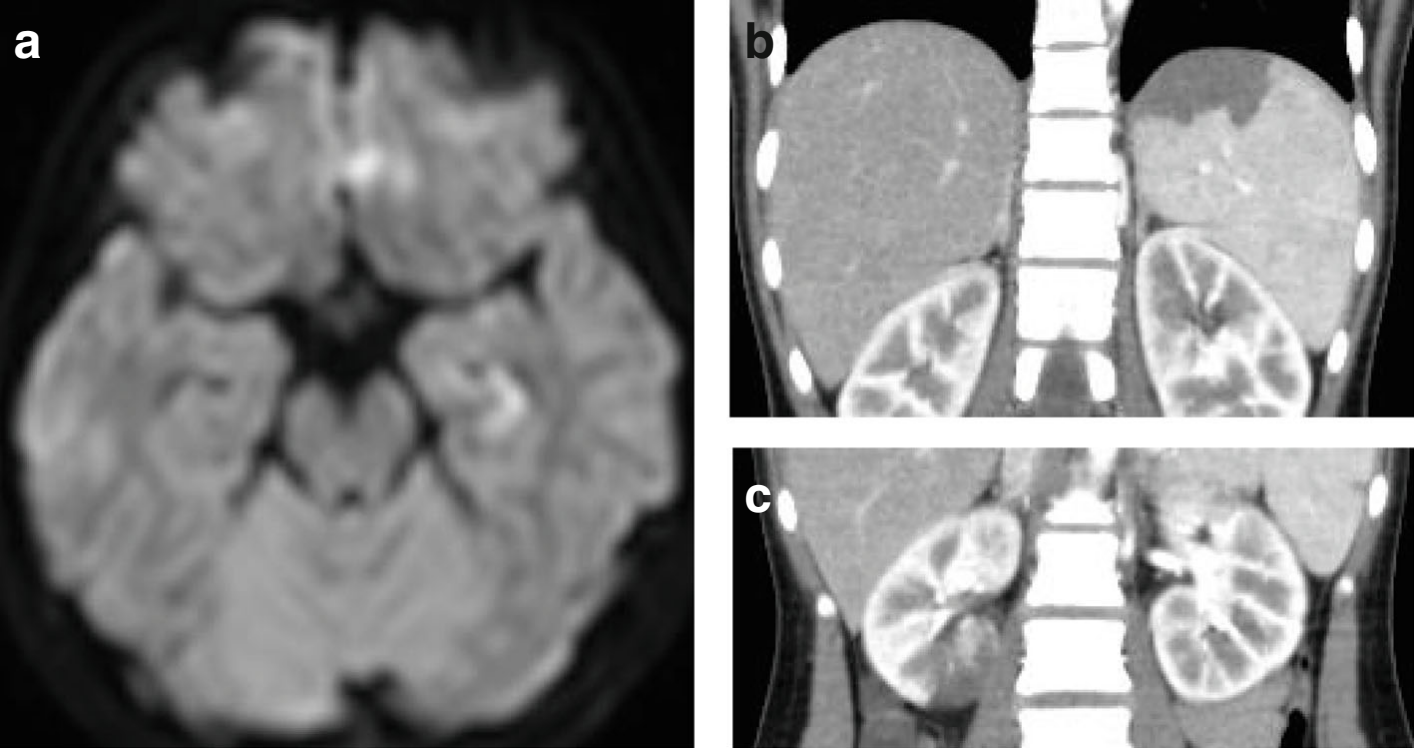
Imaging of infarction. a. Diffusion-weighted magnetic resonance imaging demonstrating infarction in deep left temporal cortex. b., c. Contrast enhanced computed tomography demonstrating infarction of spleen (b) and kidney infarction (c)
